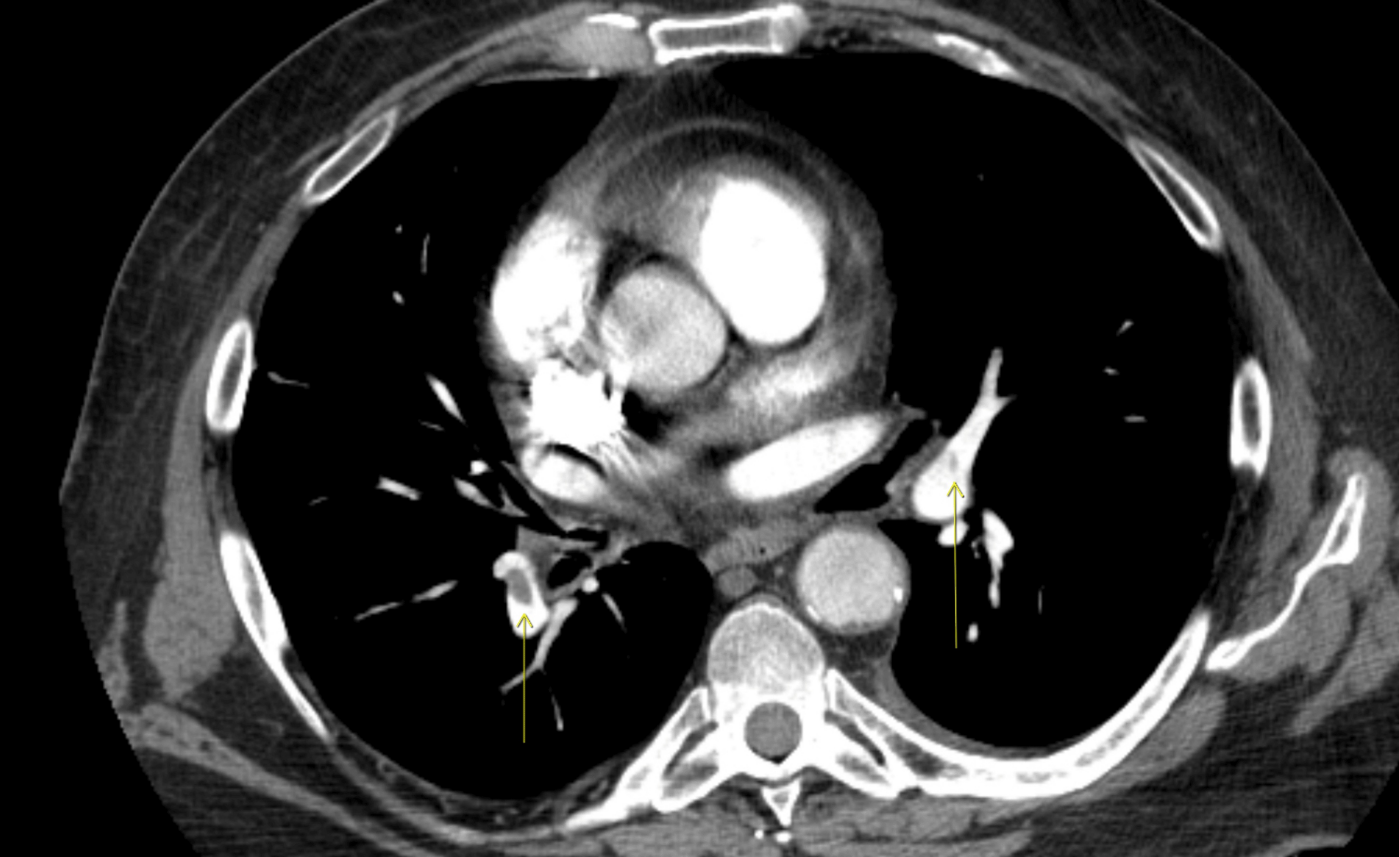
Axial CTA chest mediastinal window showing bilateral lower segmental PE (arrows) CTA, computed tomography with angiography
